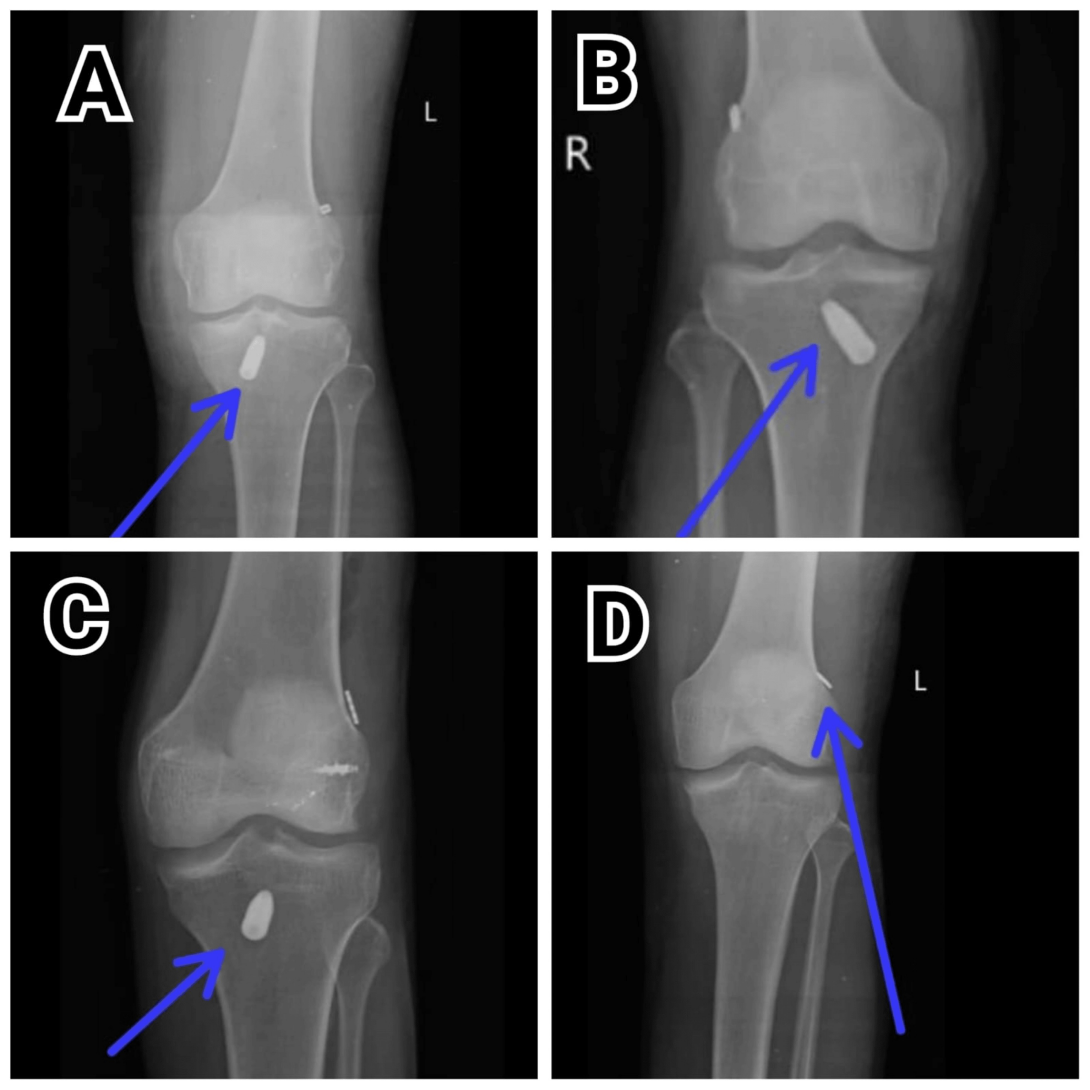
Post-operative X-rays of all the four patients A: Patient 1; B: Patient 2; C: Patient 3; D: Patient 4 The anteroposterior (AP) view of the X-ray shows the reconstruction of the anterior cruciate ligament.
